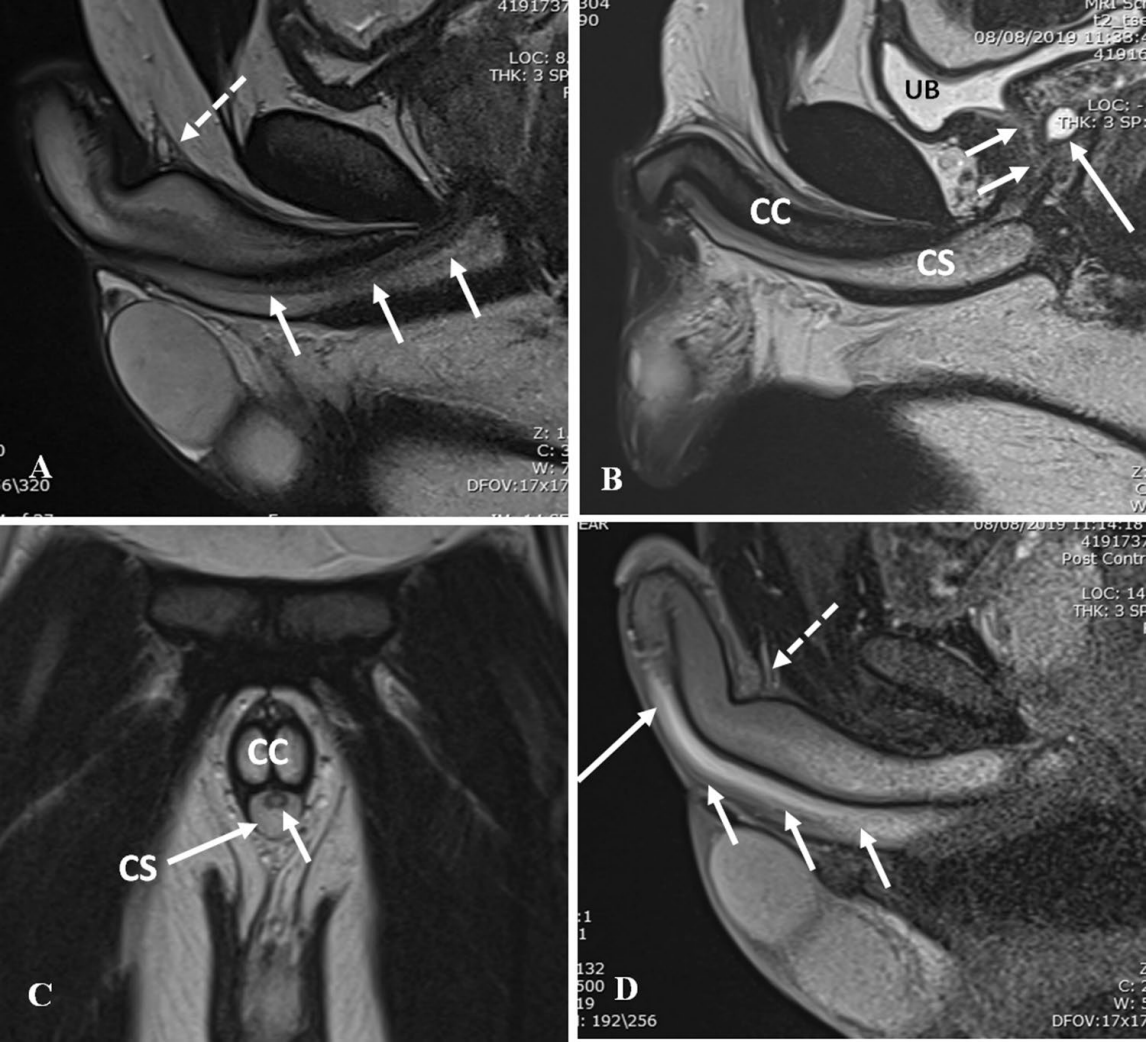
Normal urethral anatomy on MRI. a Sagittal T2-weighted MRI shows the bulbar and penile urethra (arrows). Suspensory ligament is shown inserting along the dorsal aspect of anterior urethra (dashed arrows) dividing the fixed and mobile segments of anterior urethra. b Sagittal T2-weighted MRI shows the prostatic urethra (short arrows) with incidental prostatic utricle cyst (long arrows). *CS* corpus spongiosum, *CC* corpus cavernosa, *UB* urinary bladder. c Coronal T2-weighted MRI shows the penile urethra (short arrow) lying within the corpus spongiosum (CS). *CC* corpus cavernosa. d Sagittal post-contrast T1-weighted MRI shows the enhancement along the bulbar urethra (short arrows) and penile urethra (long arrow). Suspensory ligament is shown inserting along the dorsal aspect of anterior urethra (dashed arrows)
